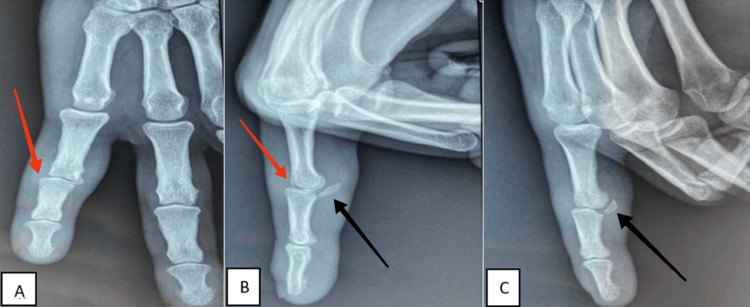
Standard radiographs anteroposterior (A), lateral (B) and lateral 3/4 (C) showing a dorsal subluxation of the PPI of the fifth right finger (red arrows) with bone avulsion of the base of the middle phalanx (black arrows).
